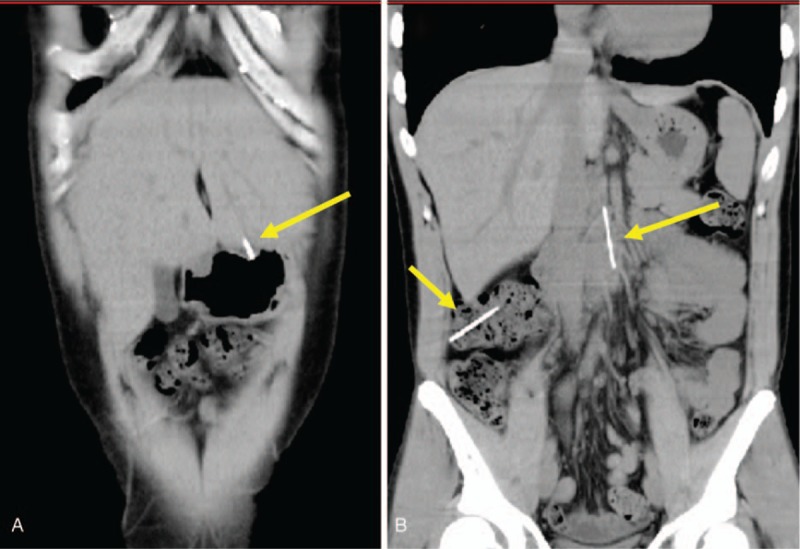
Computed tomography of the abdomen. (A) One needle was stuck in the left liver lobe through the stomach wall (yellow arrow). (B) Other needles were located in the third portion of the duodenum and transverse colon (yellow arrows).
